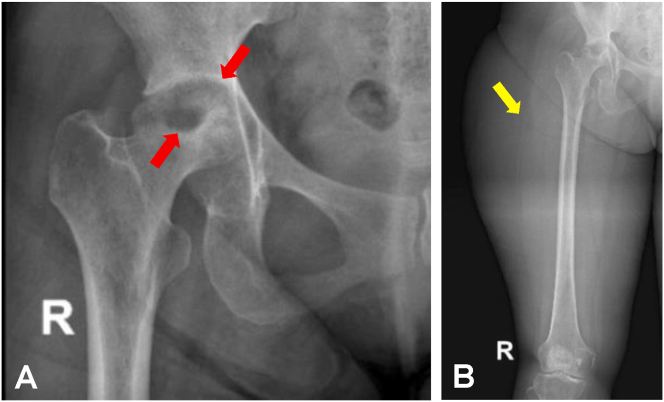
Follow-up right hip (A) dan femoral (B) radiograph after six months of anti-tuberculosis therapy. There was remaining disorganization of the hip joint with a reparative sclerotic area (red arrows) and significant reduction of soft tissue mass at the proximal thigh (yellow arrow). There is no progressive joint destruction.
